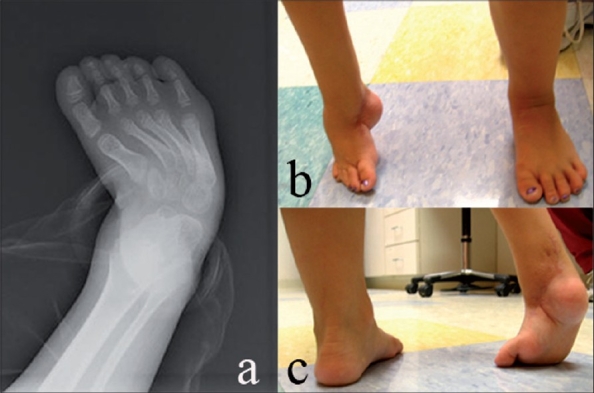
A five-year-old child with recurrent right clubfoot, depicting preoperative deformity. (a) AP X-ray of right foot with ankle showing forefoot adduction. (b,c) Clinical appearance of right foot showing forefoot supination adduction and hind foot equinus
